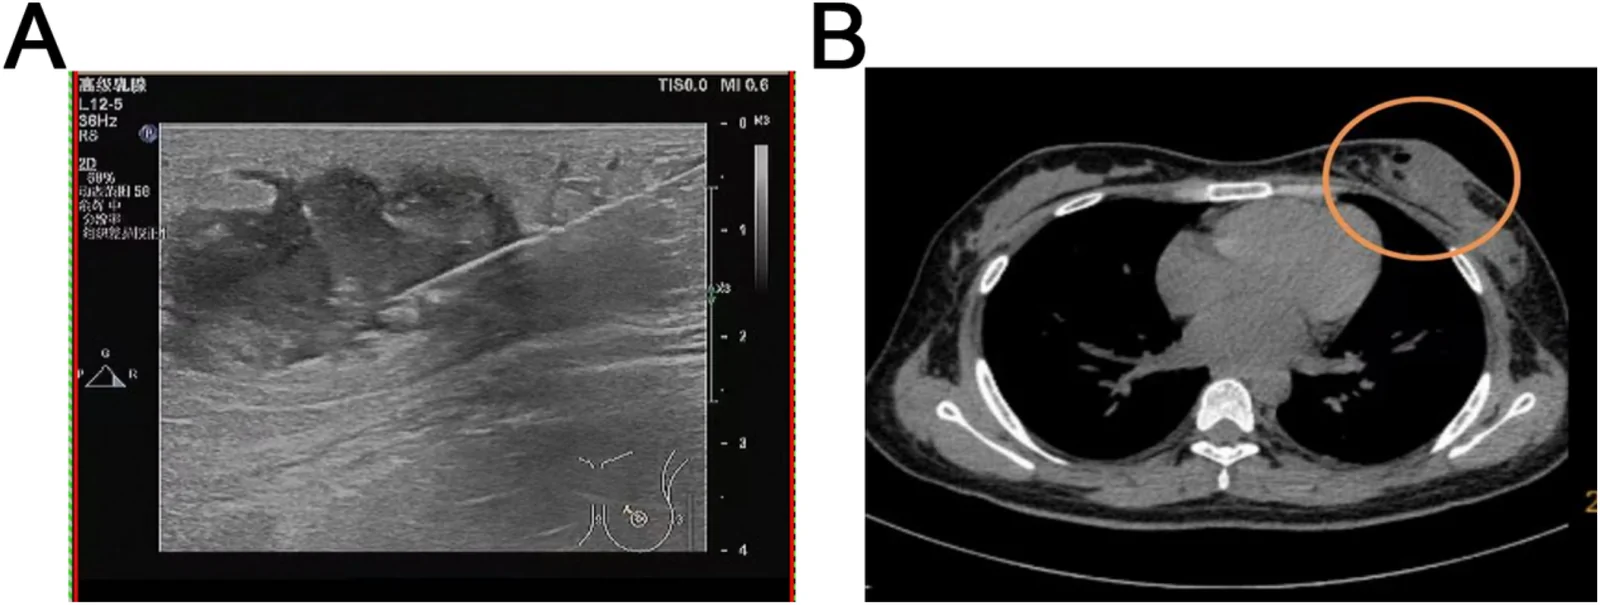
Radiological findings of the left breast lesion. (A) Breast ultrasound demonstrating a mixed echogenic lesion measuring approximately 5.1 × 5.0 × 2.3 cm with ill-defined borders, irregular shape, and heterogeneous internal echoes, suggestive of mastitis with abscess formation (BI-RADS category 3). Left axillary lymphadenopathy was also noted. (B) Chest computed tomography (CT) showing enlargement of the left breast with multiple scattered punctate gas densities (arrows) in the medial aspect, with surrounding fat stranding and left axillary lymphadenopathy, consistent with suppurative mastitis.
